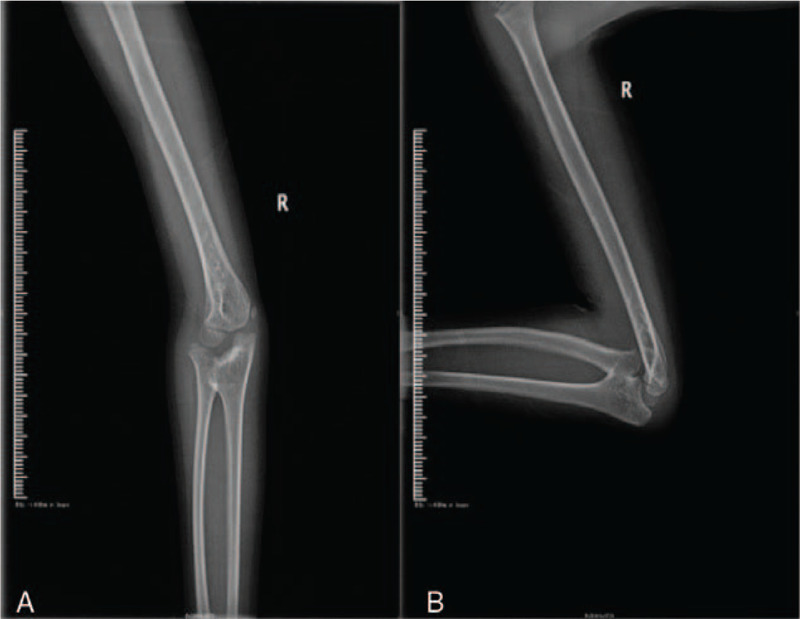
(A) Preoperative X-ray of the anteroposterior elbow. (B) Preoperative X-ray of the lateral elbow.
